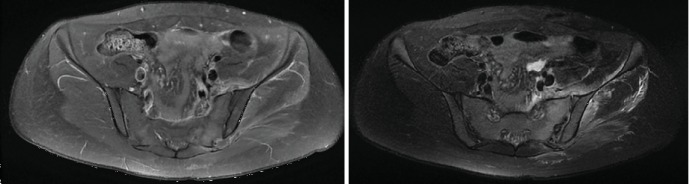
MRI of the pelvis: T1-enhanced and T2 axial views showing signal abnormality of the left sacroiliac joint, predominant in the sacral wing, characterized by hyperintensity on T2. There are significant signal abnormalities and gadolinium uptake of the musculature adjacent to the left sacroiliac joint. Anterior effusion of the left sacroiliac joint is visible with additional bulging of the posterior articular capsule.
